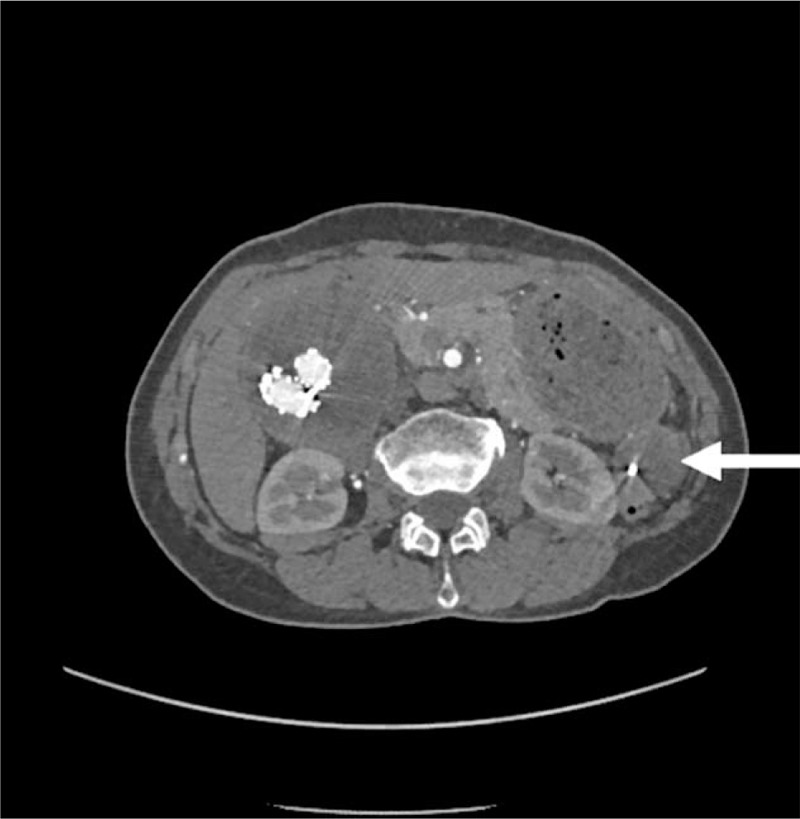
Contrast-enhanced computed tomography (CT) 2 months after surgery. There was thrombosis in the pseudoaneurysm. High-density metal shadow was seen in and around the pseudoaneurysm. There was no effusion in the abdominal and pelvic cavity.
